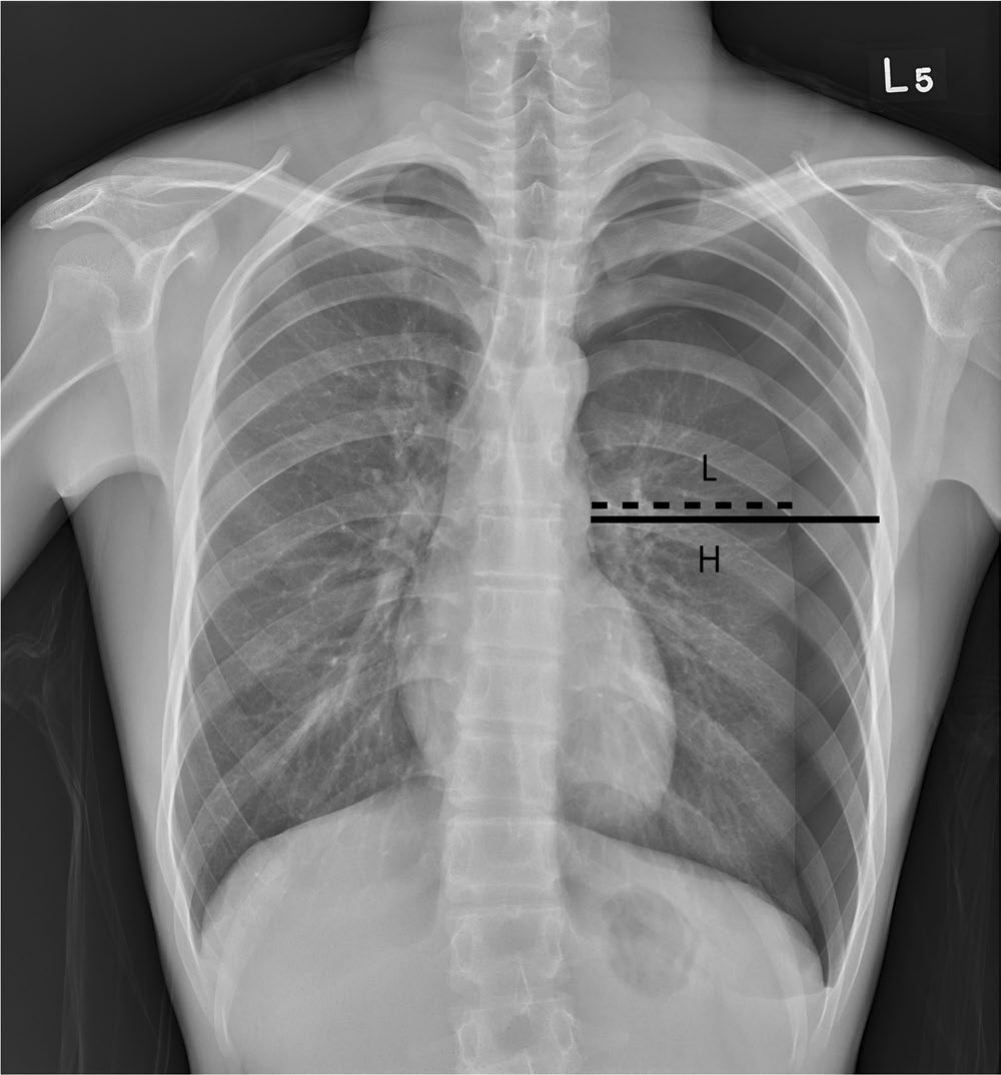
Chest radiograph of a 17-year-old boy with a primary pneumothorax. H represents the diameter of the inner hemithorax at the hilar level, and L represents the diameter of the collapsed lung. Using the Light equation: the size of pneumothorax = (1 − L^3^/H^3^) × 100%, we can easily calculate an estimated pneumothorax size of 34%.
